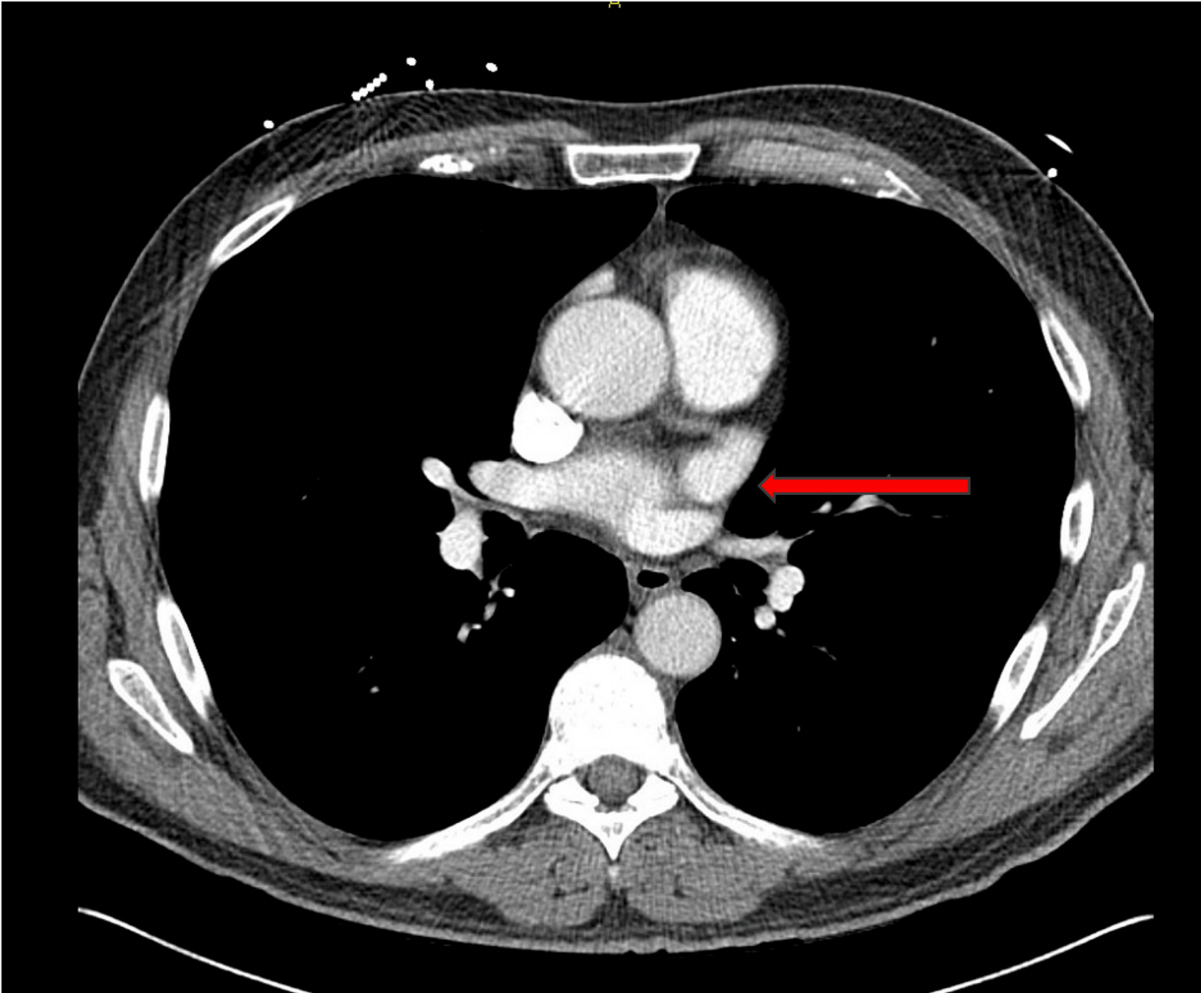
Chest CT showing an enlarged multistation intrathoracic lymph nodes with enlarged right lower paratracheal lymph node, prominent para-aortic lymph node, and a left internal mammary nodal mass concerning for nodal metastasis (red arrow)
